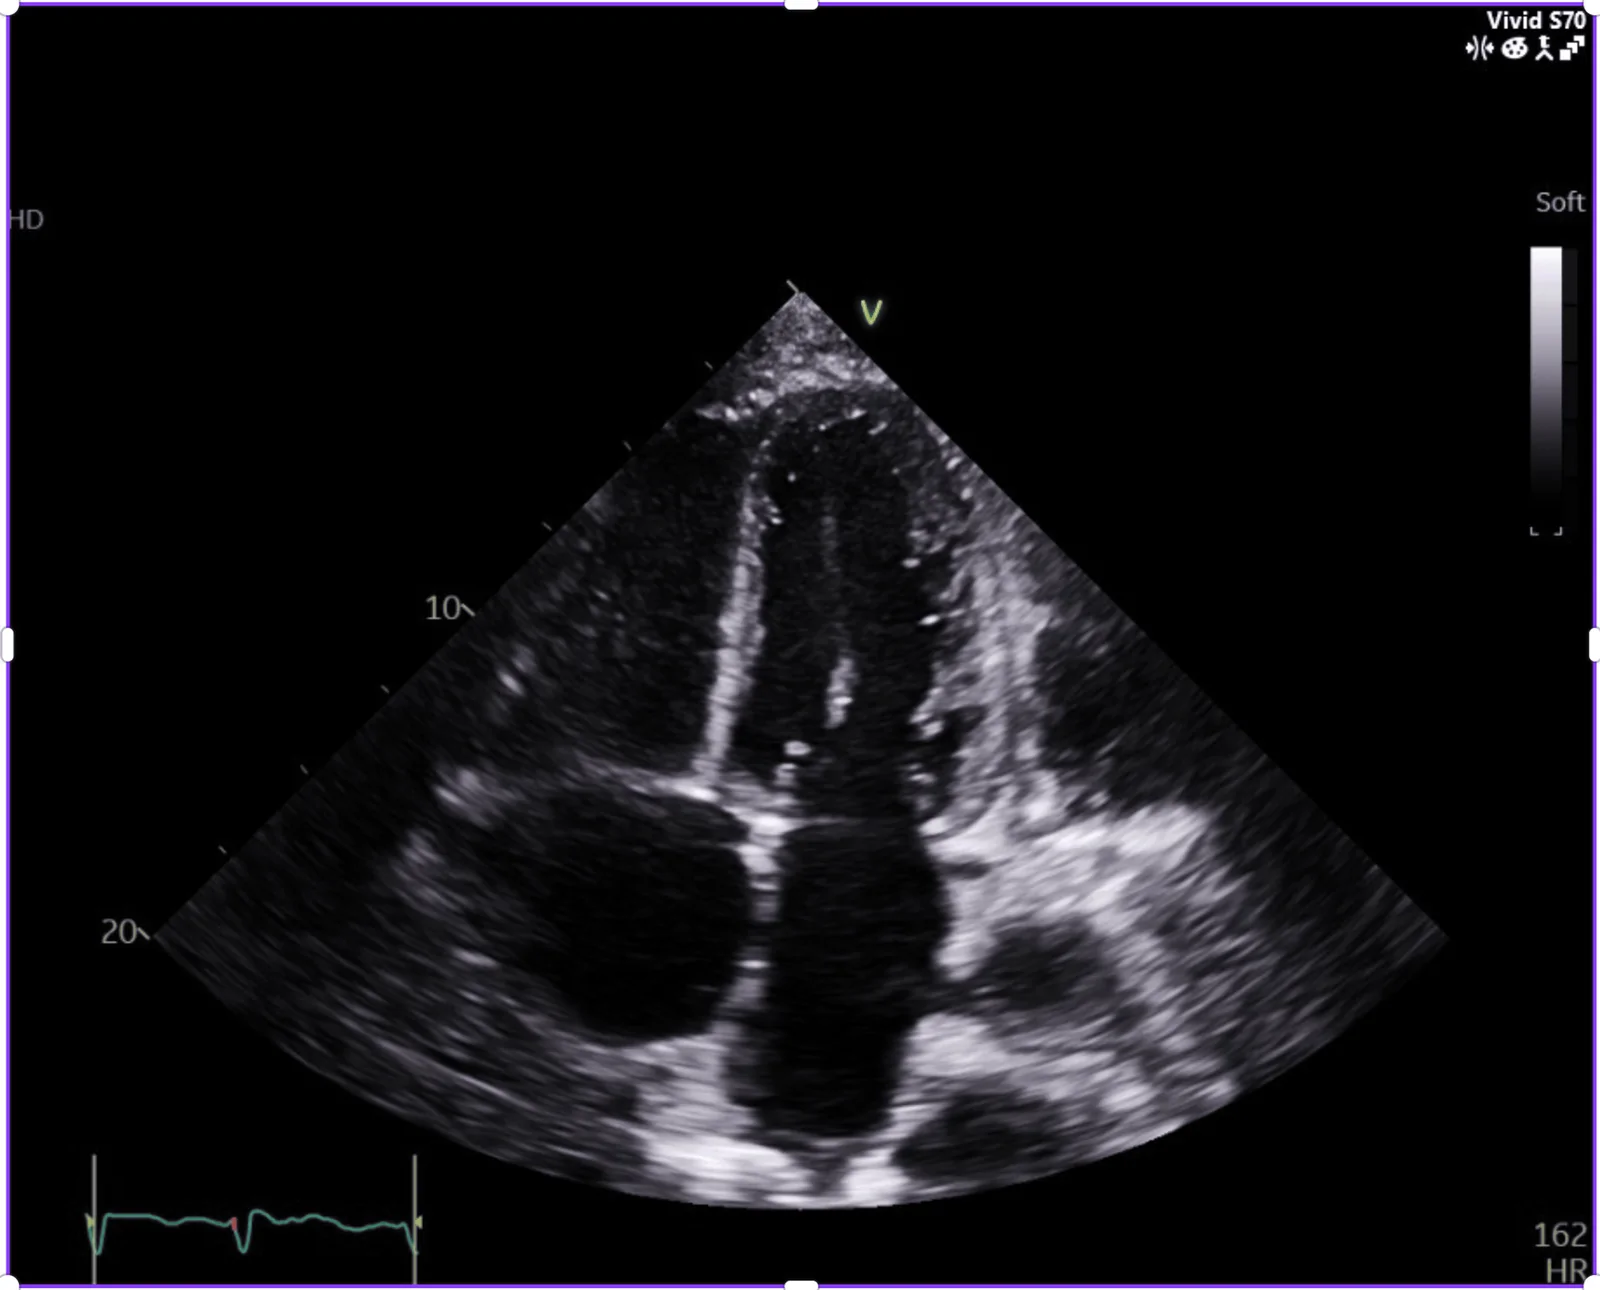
Apical four-chamber transthoracic echocardiogram at presentation, obtained during diastole phase, demonstrating a dilated left ventricle with severe biatrial dilatation.
